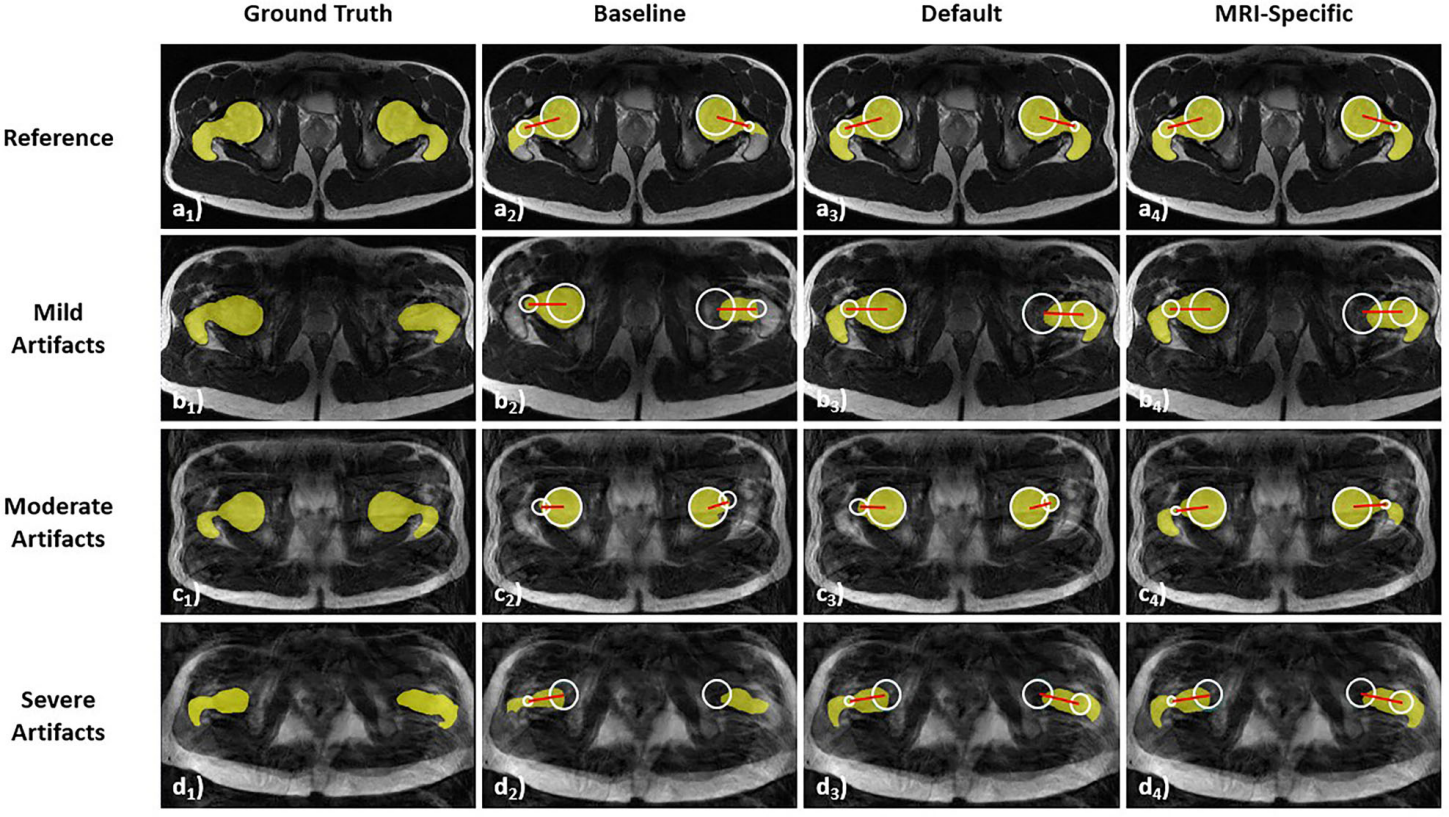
Segmentation quality as a function of augmentation strategy and artifact severity. MR images with overlaid segmentation outlines of the hip are shown. The reference image is unaffected by artifacts, while the other images are affected by varying degrees of artifact severity, from mild to severe. Color coding and image overlays as in Fig. 1. Absence of reference lines (d_2_) indicates that no reference line could be computed. Improved segmentation and post hoc processing were observed with more extensive augmentation during training when fewer artifacts were present. For these images, the Dice Similarity Coefficients (DSC) were as follows. Reference image: 0.91 (a_2_), 0.96 (a_3_), 0.96 (a_4_). Image with mild artifacts: 0.58 (b_2_), 0.85 (b_3_), 0.84 (b_4_). Image with moderate artifacts: 0.51 (c_2_), 0.65 (c_3_), 0.82 (c_4_). Image with severe artifacts: 0.84 (d_2_), 0.89 (d_3_), 0.89 (d_4_). Manual and computed femoral torsional values (R/L [°]) were as follows. Reference image: 9.5/12.4 (a_1_), 12.9/12.7 (a_2_), 12.7/12.0 (a_3_), 10.6/11.8 (a_4_). Image with mild artifacts: 6.0/−0.3 (b_1_), 5.1/−0.5 (b_2_), 5.3/−0.4 (b_3_), 5.7/−0.3 (b_4_). Image with moderate artifacts: −0.9/−1.5 (c_1_), −0.1/−6.0 (c_2_), −0.1/−5.5 (c_3_), −0.9/−1.2 (c_4_). Image with severe artifacts: 10.3/12.2 (d_1_), 11.5/NA (d_2_), 11.5/16.0 (d_3_), 11.5/13.6 (d_4_)
